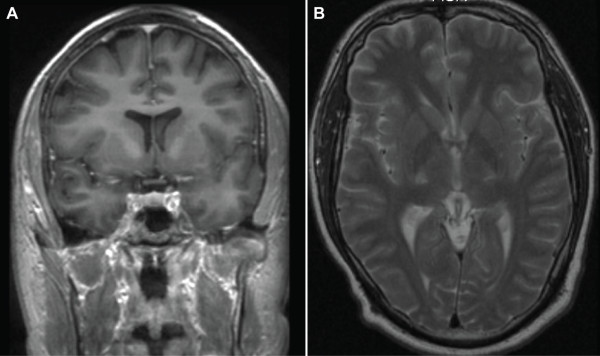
MRI of the brain in a patient with AMN showing increased signal in the pyramidal tracts on T2-weighed coronal (A) and axial (B) images indicative of Wallerian degeneration.
